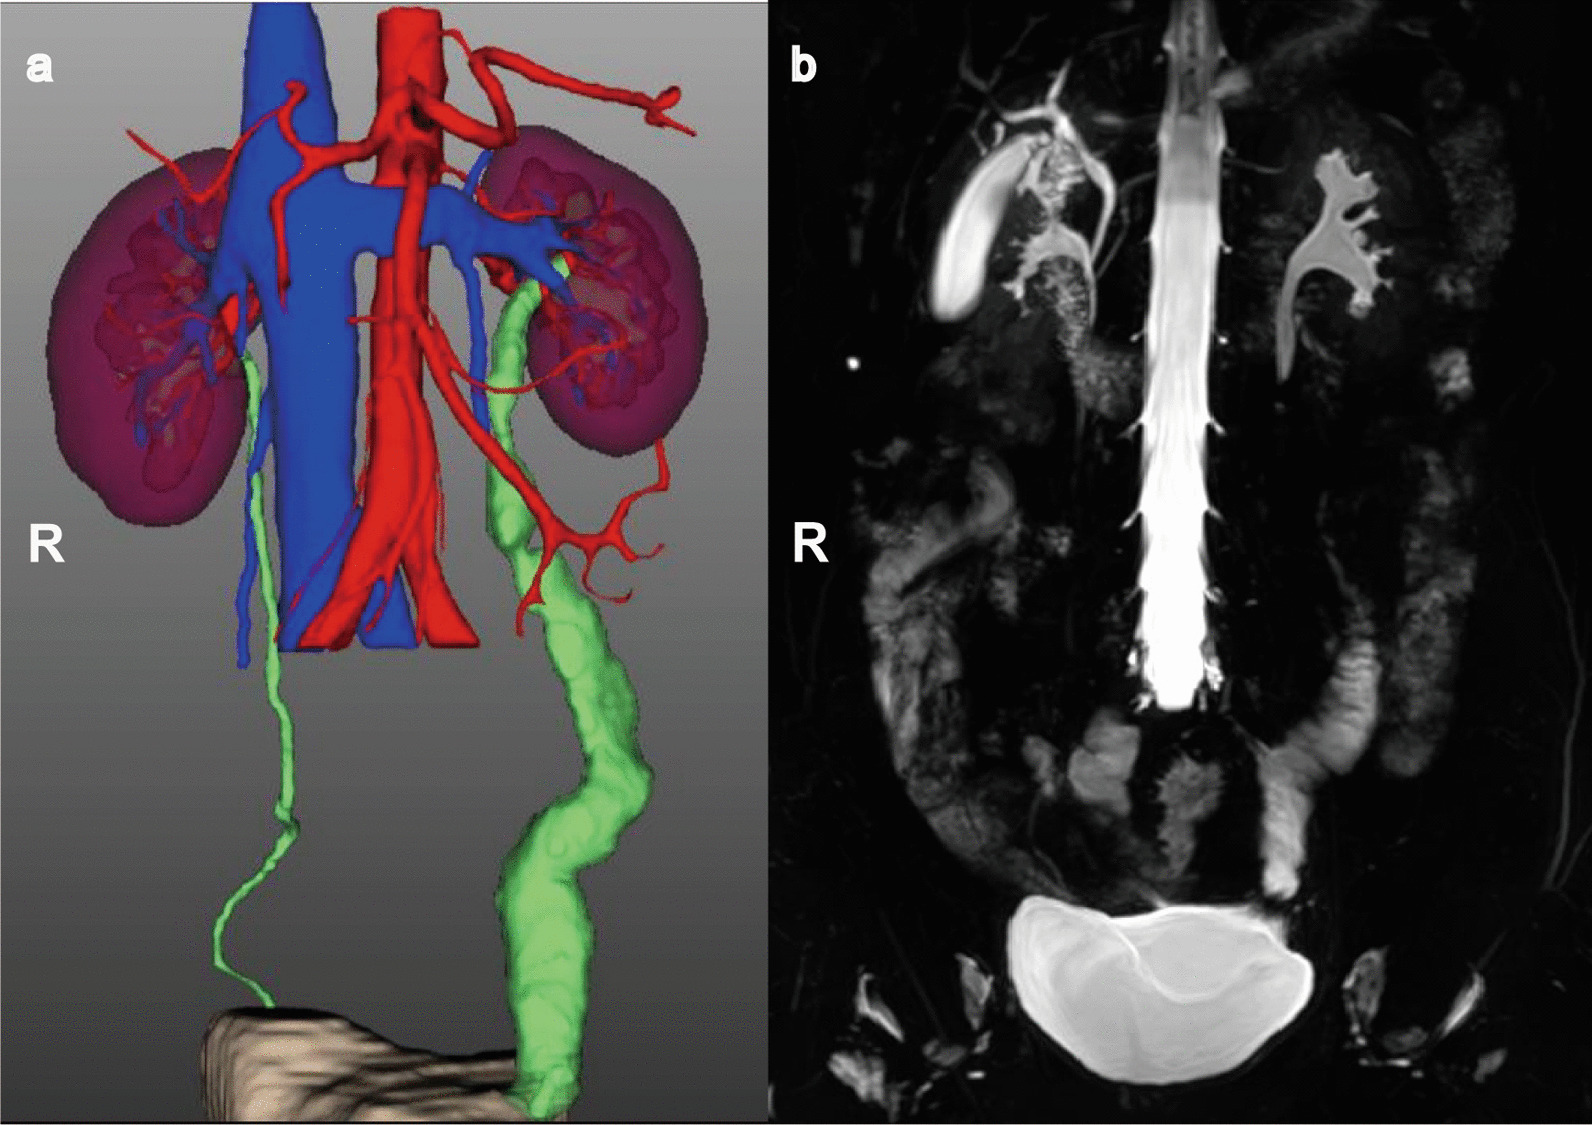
Postoperative evaluation at the follow-up. 3D CT image reconstruction (a) and magnetic resonance urography (b) demonstrate the morphology of ileal ureter replacement without hydronephrosis
